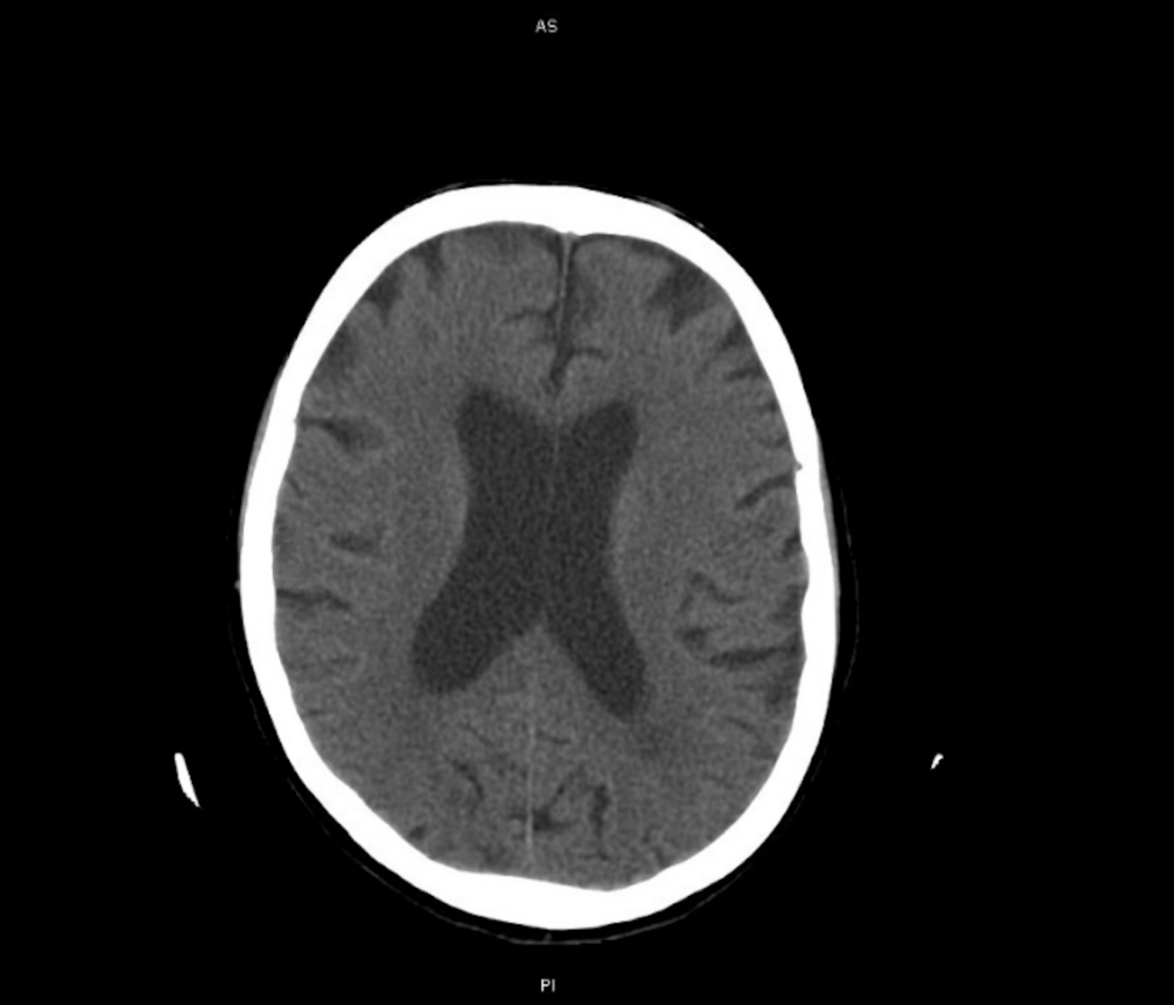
CT demonstrating a normal scan with no acute findings.
